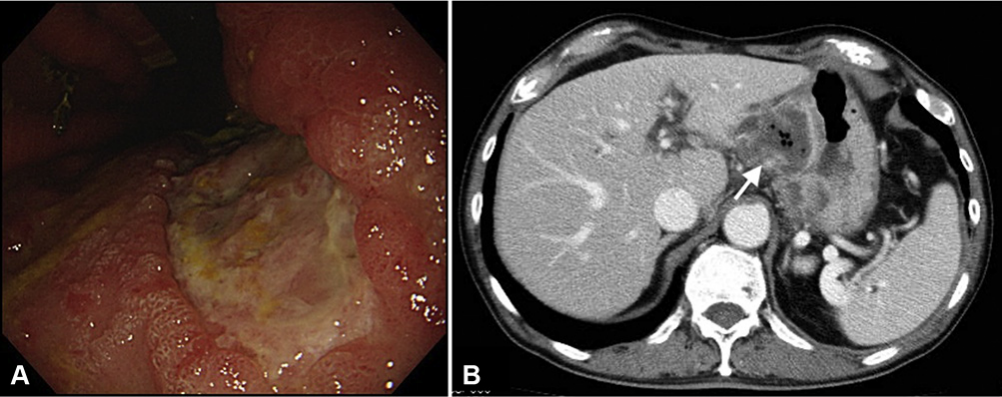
Follow-up endoscopic and CT findings on postoperative day 12. A, The perforation was closed. B, The intra-abdominal abscess (*white arrow*) is still present.
